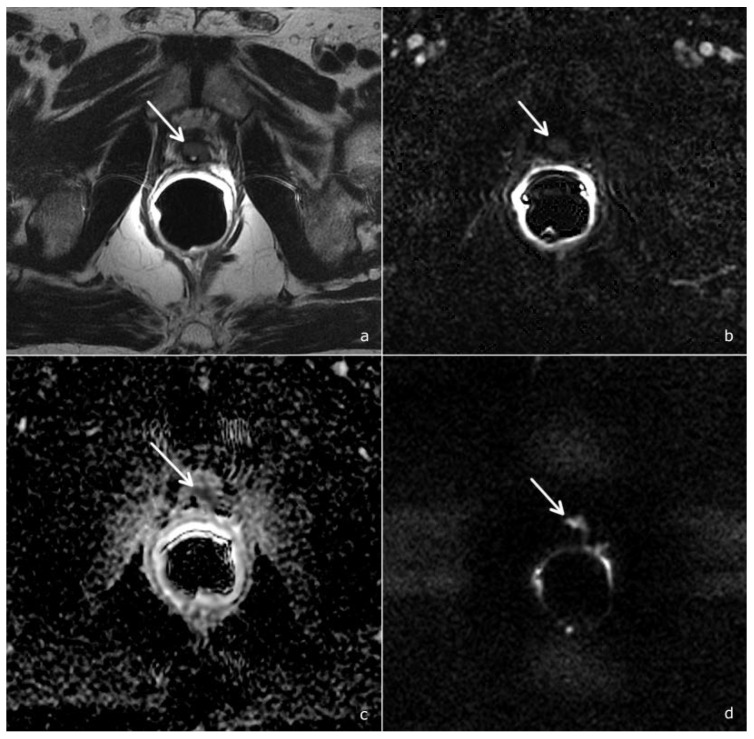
A PI-RR-score-4 (arrows), in a patient 72 years old, with a PSA value of 0.71 ng/mL. (a) T2-weighted image documents hypointense tissue localized anteriorly to the vesico-urethral anastomosis. (b) DCE depicts a focal mild and late enhancement. However, as the ADC map (c) and DWI (d) show a significant diffusion restriction (DWI score = 4), the overall PI-RR score was 4.
